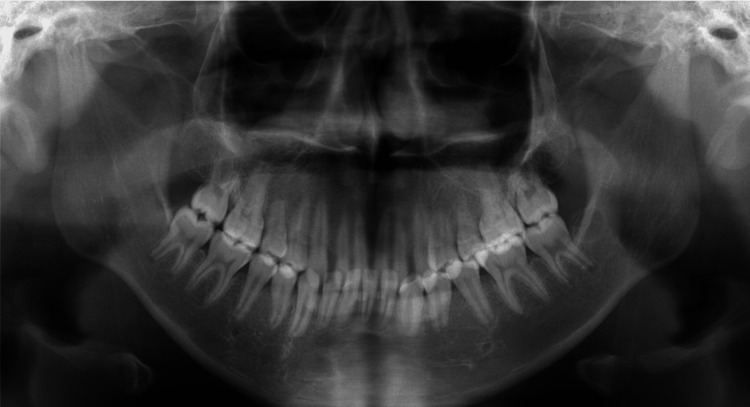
Pretreatment evaluation, Panoramic radiograph.
